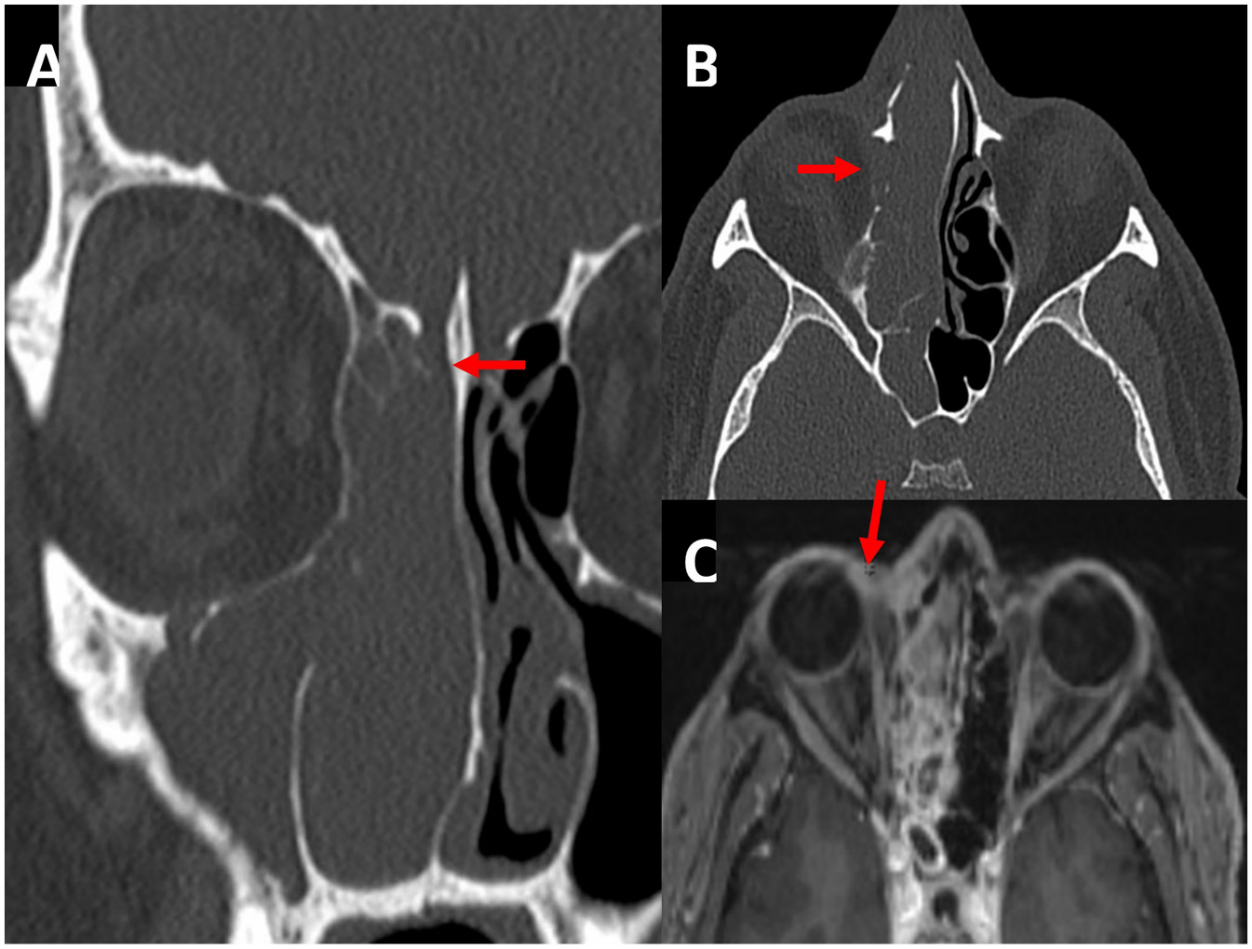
Computed tomography demonstrating potential thinning at the right cribriform plate in (A, red arrow) versus volume averaging and demineralization of the lamina papyracea with concern for extension of disease into the periorbital tissue (B, red arrow). Axial T1 postcontrasted magnetic resonance image slice depicting soft tissue involvement abutting the lacrimal sac (C, red arrow).
